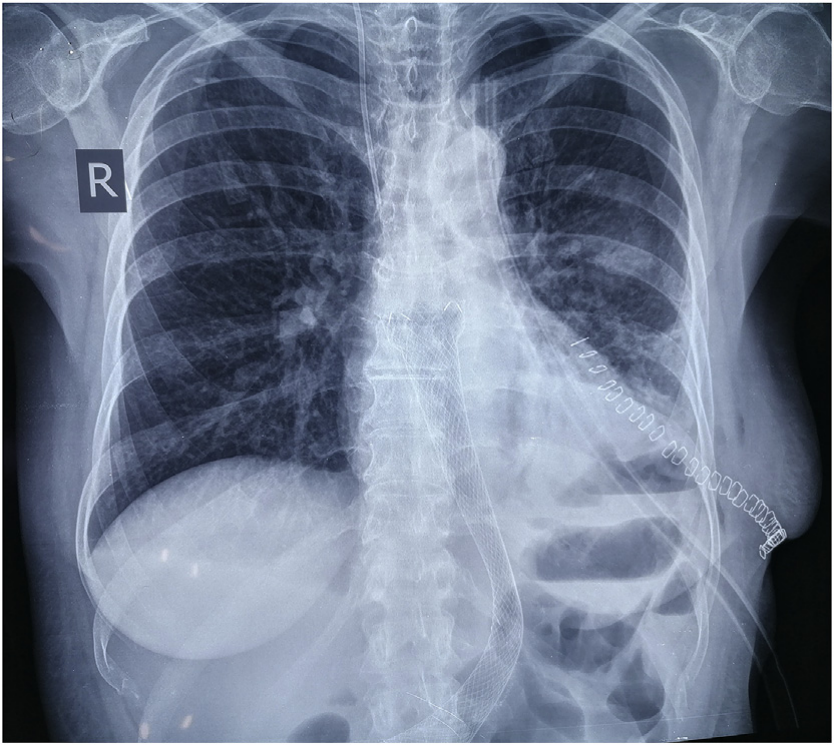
Post-operative CXR showing full lung expansion, the stent in its place, the postoperative chest X-ray and the metallic skin clips.
